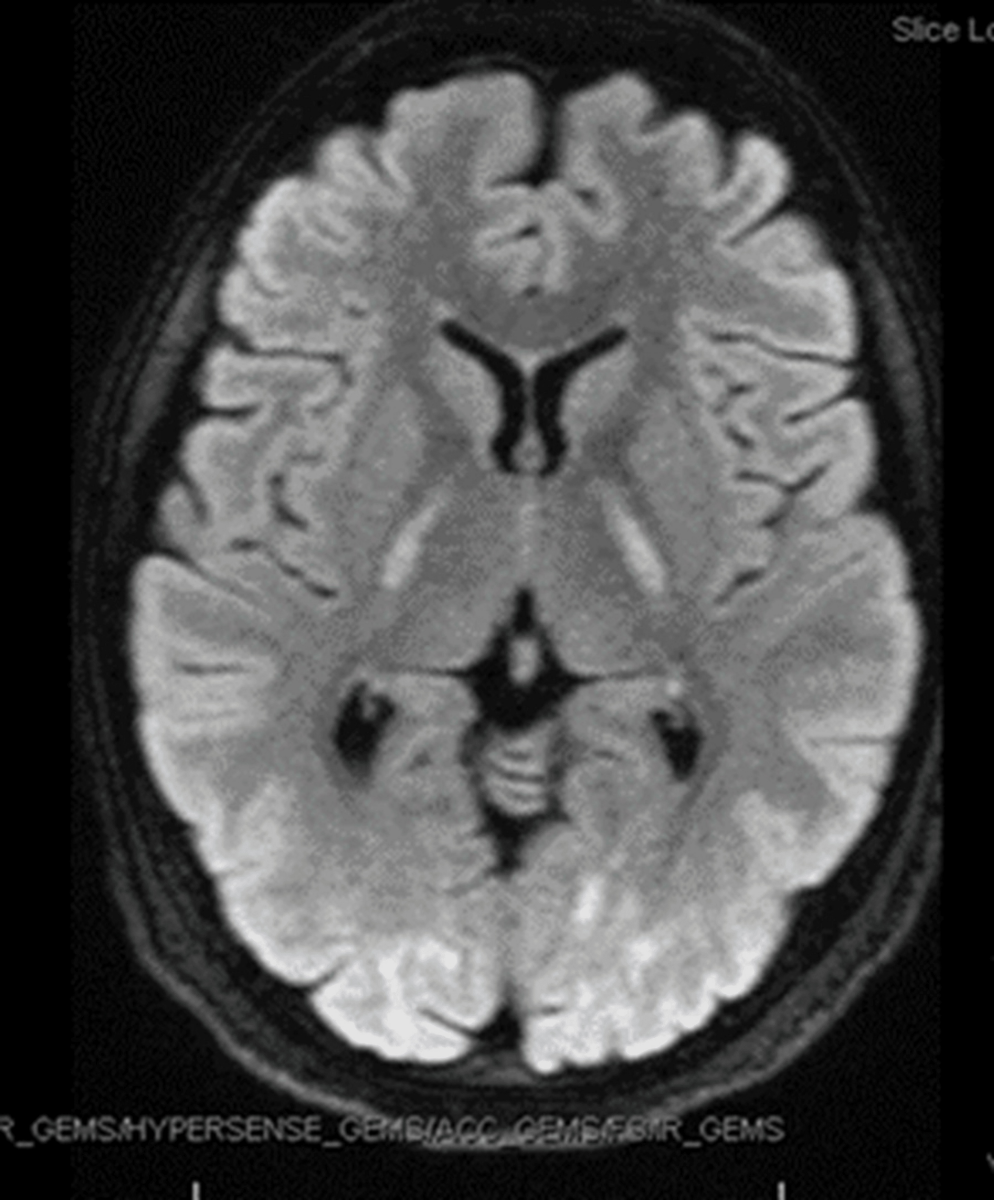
Flair axial view showing hyperintensities in the occipital lobe and the internal capsule FLAIR: fluid-attenuated inversion recovery
